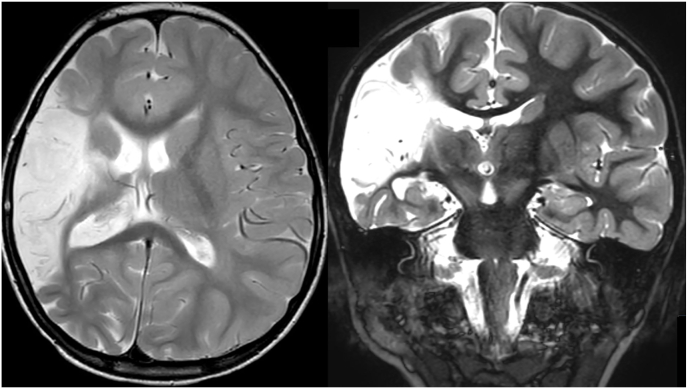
MCA-Infarct: 4yrs old male, delayed motor development, refractory seizures for 18 months; hemiparesis left, able to walk. T2 weighted MRI transversal (left) and coronar (right): Perinatal MCA-infarction involving the pyramidal tract.
